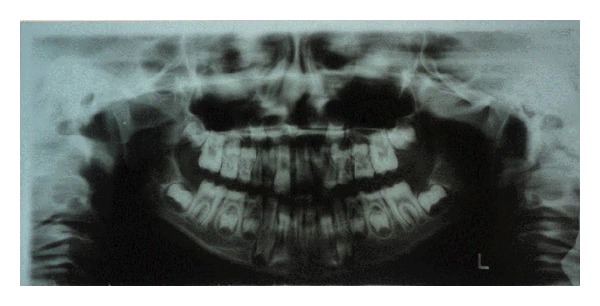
Panoramic radiograph showing that the thin enamel layer could not be distinguished from the underlying dentin.
